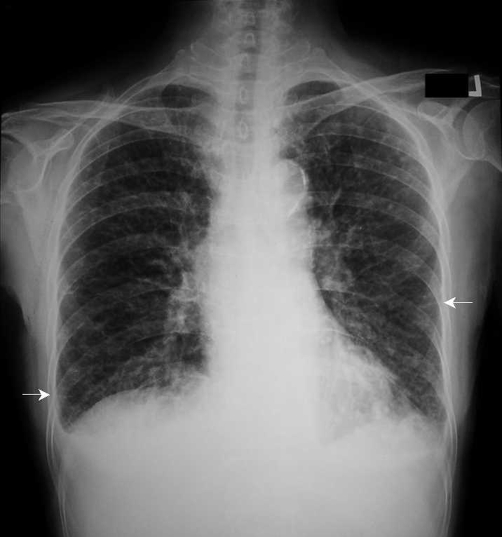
Chest radiograph showing diffuse interstitial pulmonary infiltrates with Kerley B lines (arrows) and pleural effusion.
